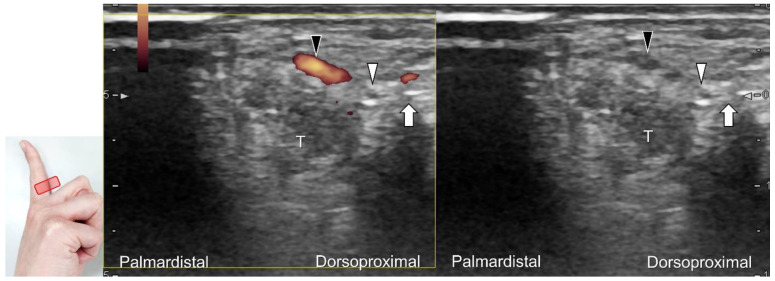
Ultrasound-guided injection to the dorsal proper digital nerve in its short axis with the dual imaging mode (Doppler vs. B mode). White arrowhead: dorsal proper digital nerve; black arrowhead: dorsal proper digital artery; arrow: needle. T: flexor tendons.
